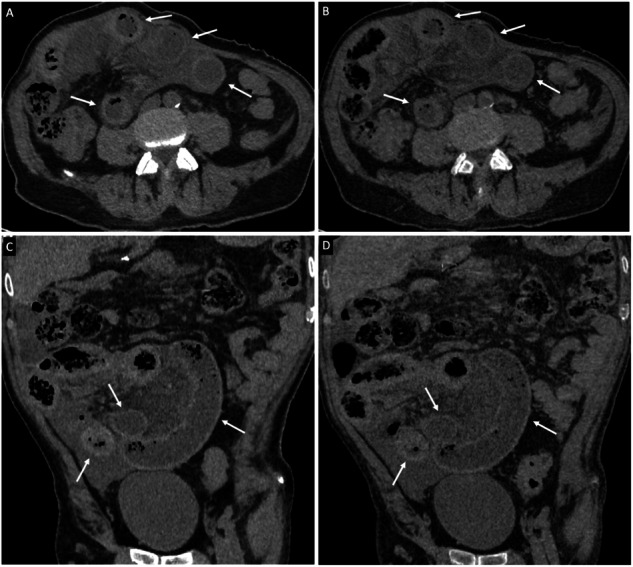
CT images in a 71-year-old man with small-bowel ischemia resulting from closed-loop small-bowel obstruction. The patient was treated by surgery and underwent bowel resection. (A, B) Axial and (C, D) coronal; (A, C) TUE and (B, D) VUE images show increased mural attenuation of the involved loops (arrows)
